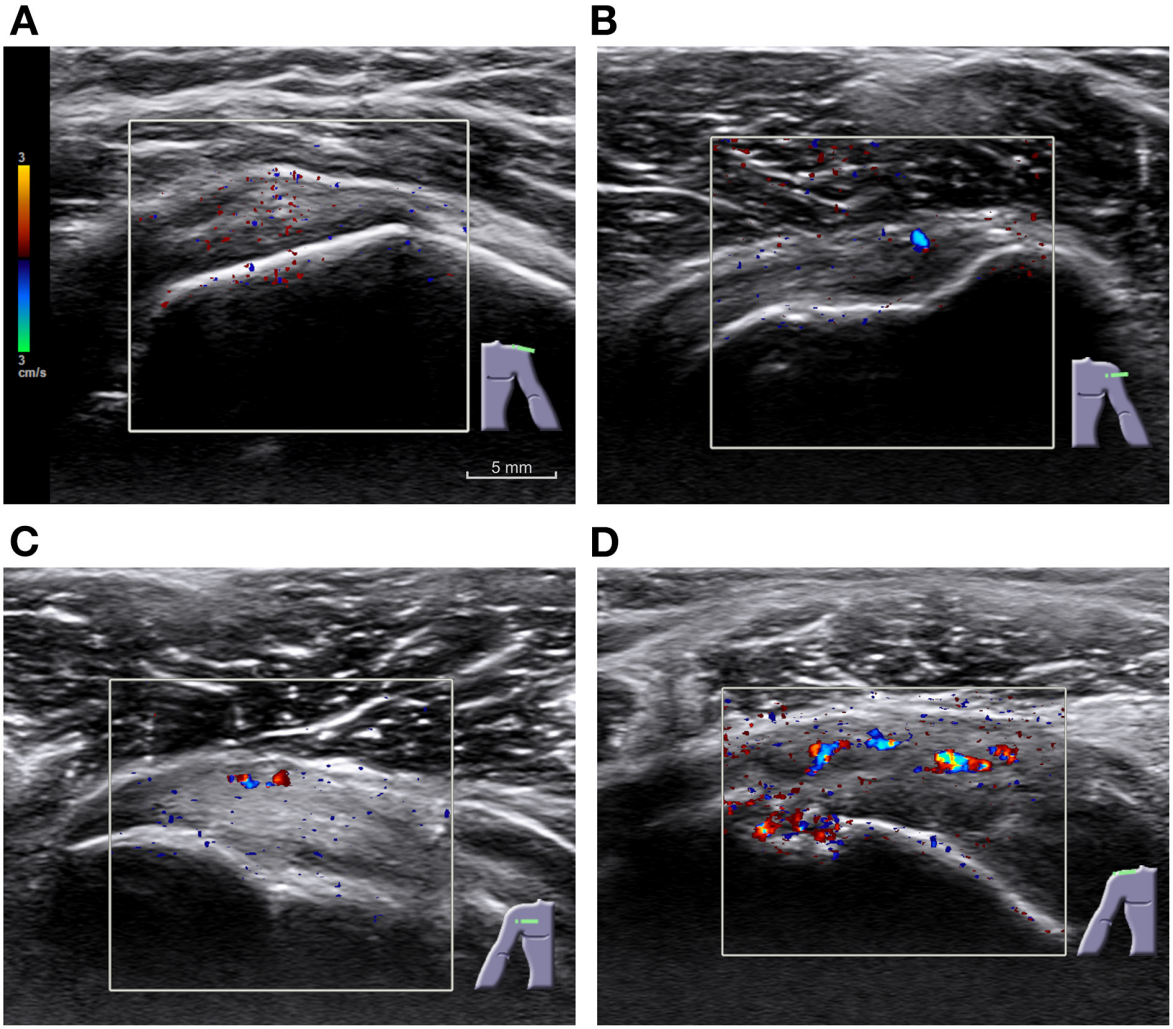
Examples of different grades of Color Doppler in rotator cuff tendons of symptomatic participants. (A) Grade 0, with no significative signal and only minimal scattered noise; (B) grade 1, with one or two points or short lines; (C) grade 2, showing three to six points or short lines; and (D) grade 3, with more than six points, a continuous line, or a bar. A pulse repetition frequency (PRF) of 488 Hz was used. Scale and color coding are identical across images.
